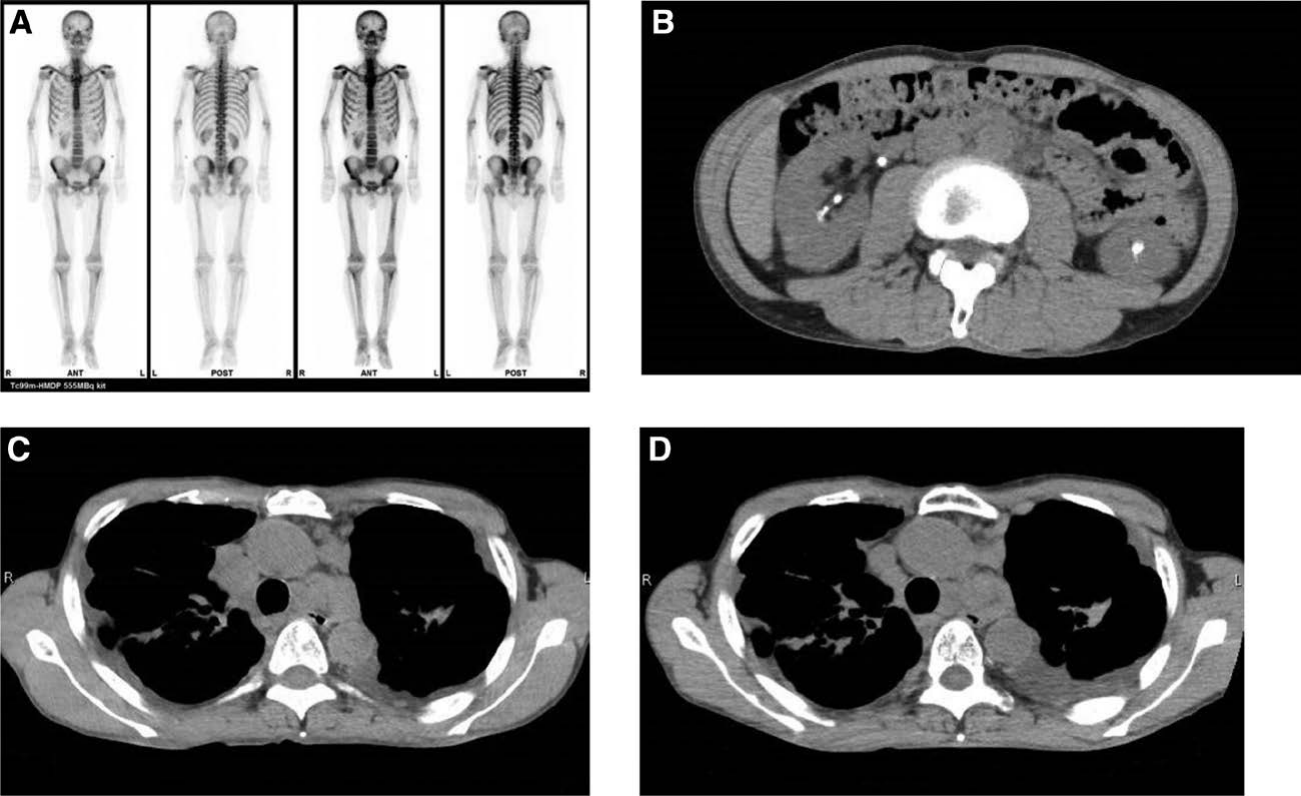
Imaging studies in the present case. (A) Bone scintigraphy showed no sign of bone malignancy. (B) Computed tomography of the abdomen taken at admission. Multiple nephrolithiasises were observed in both kidneys without evidence of hydronephrosis. (C and D) Computed tomography of the chest taken 6 months before admission (C) and at admission (D). Bilateral hilar lymphadenopathy shows no apparent size changes over the past 6 months.
